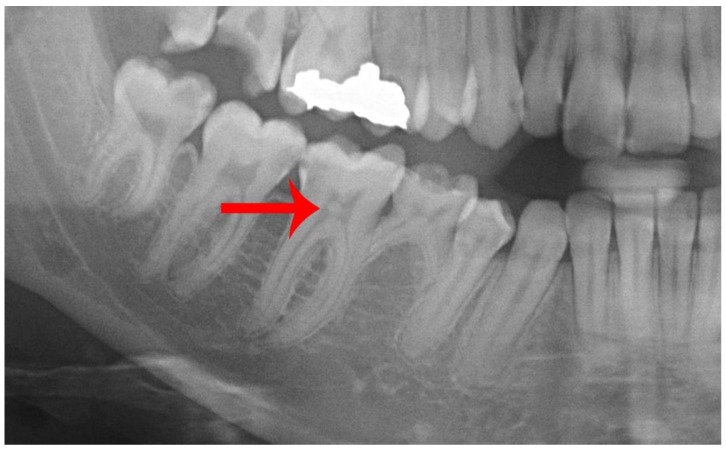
Close-up view of a panoramic radiograph showing idiopathic pulp stone within the coronal pulp chamber of the lower right first molar (Red arrow).
